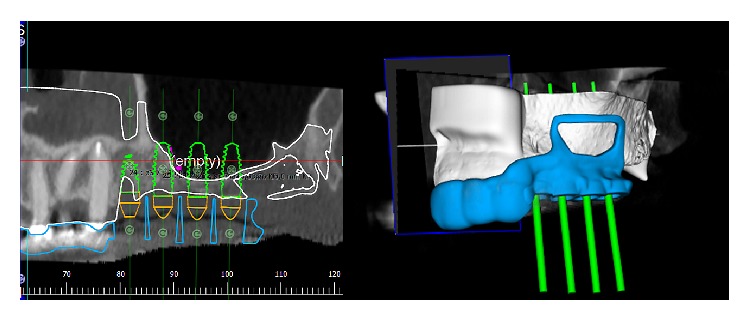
The position of the implants is preplanned on the CBCT scan. A surgical guide is designed that includes also the frame of the sinus antrostomy designed according to the low window principles.
